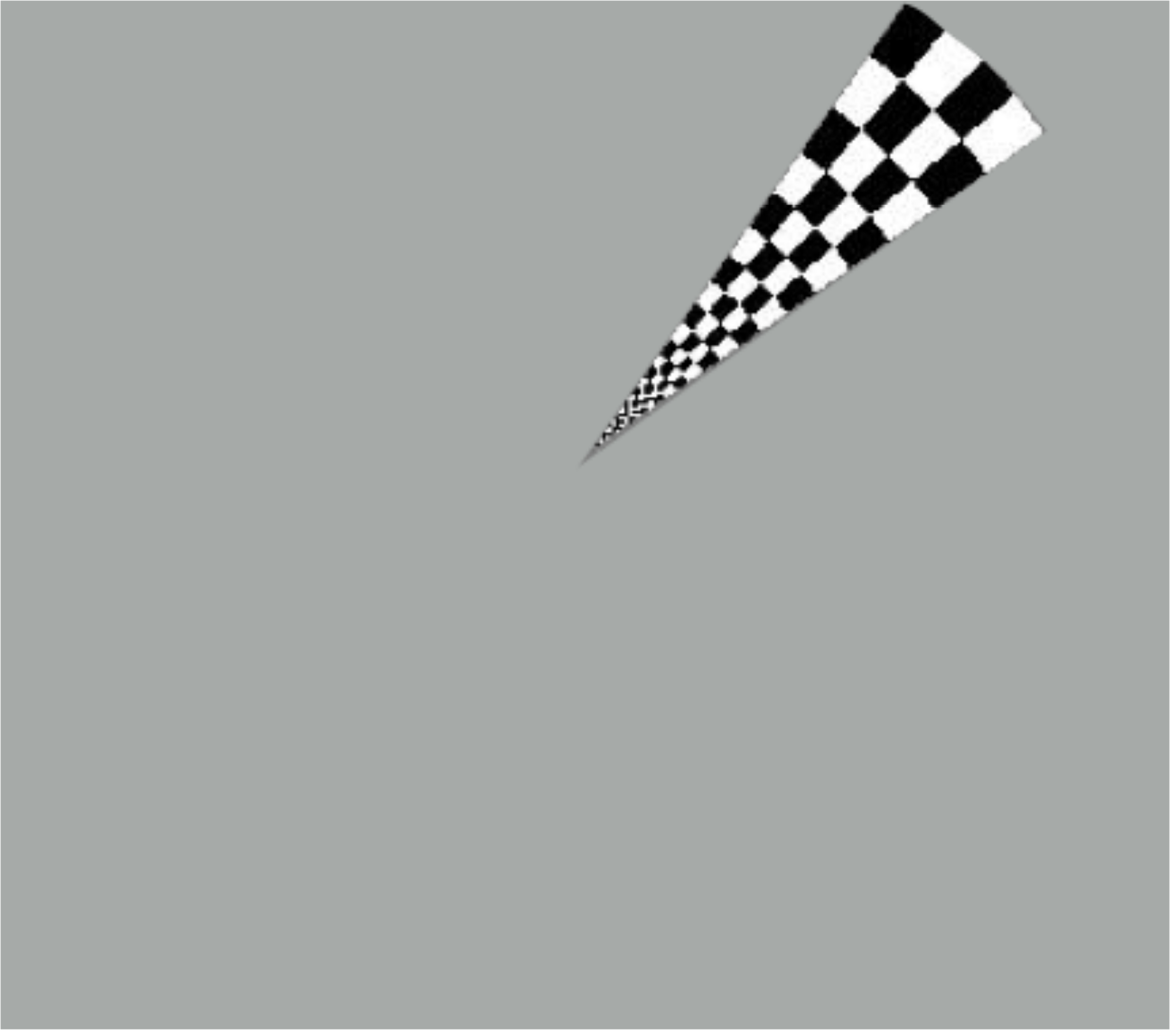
Polar angle visual stimuli used during functional magnetic resonance imaging scanning.
